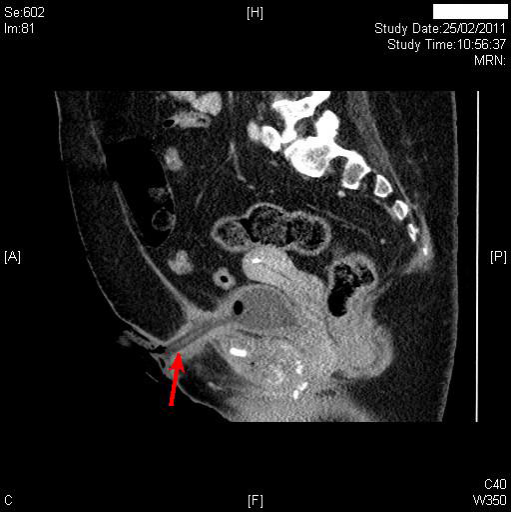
Sagittal reconstruction of Computed tomography, performed eight months after first CT, revealed persistent soft tissue thickening along the tract of the suprapubic catheter with mild localised inflammation in the abdominal wall fat (red arrow). This soft tissue thickening showed some enhancement suggesting chronic inflammation.
